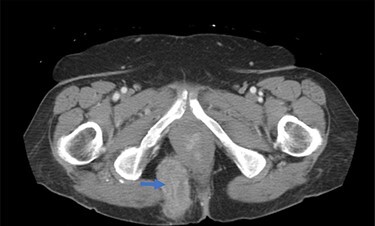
Preoperative CT demonstrating pararectal hernia (blue arrow).
